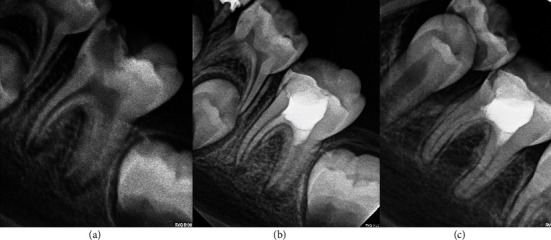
Permanent tooth 36. The image (a) shows diagnostic X-ray, image (b) immediate postoperative, and image (c) control at 79 months.
